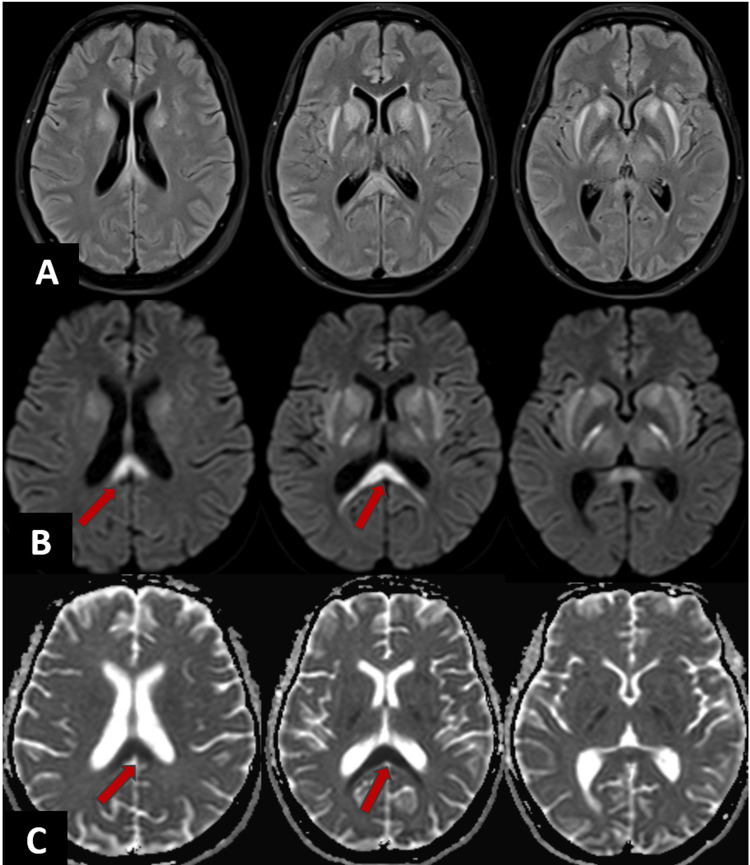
Extrapontine involvement and associated CLOCC Axial FLAIR image (A) demonstrates symmetric hyperintensities involving the caudate nuclei, putamina, thalami, internal and external capsules, hippocampi, and cerebellar hemispheres. Axial DWI (B) and ADC map (C) demonstrate mild diffusion restriction limited to the capsular regions. A focal splenial lesion demonstrates marked diffusion restriction on DWI with corresponding low ADC values, consistent with a CLOCC (red arrows). CLOCC: cytotoxic lesion of the corpus callosum; DWI: diffusion-weighted imaging; ADC: apparent diffusion coefficient; FLAIR: fluid-attenuated inversion recovery
